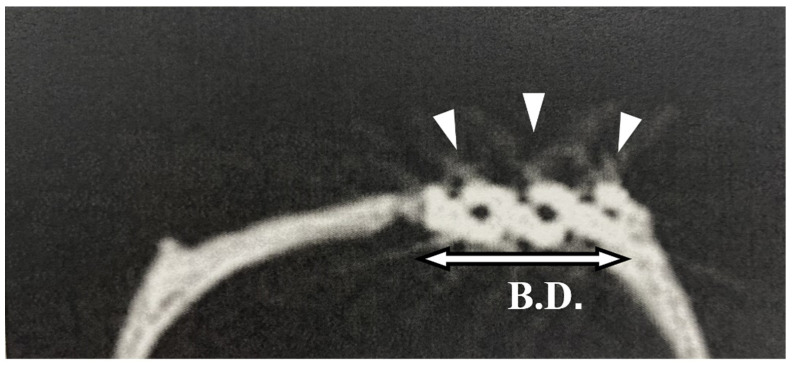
Micro-computed tomography image of a placed implant. The porous additive-manufacturing titanium artificial bone (pAMTAB) is observed in the calvarial bone defect; there is no deviation. pAMTAB is denoted by arrow heads.
